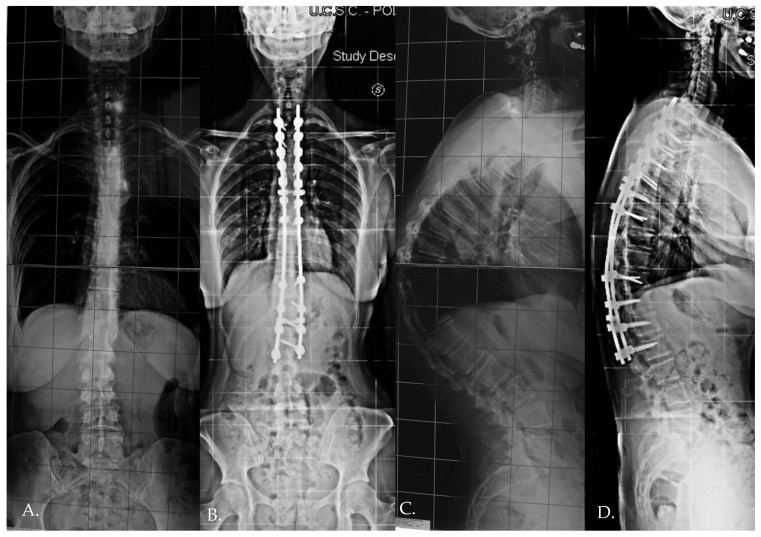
Standing preoperative (A,C) X-rays and immediate postoperative X-rays (B,D) of a 23 years old female patient.
